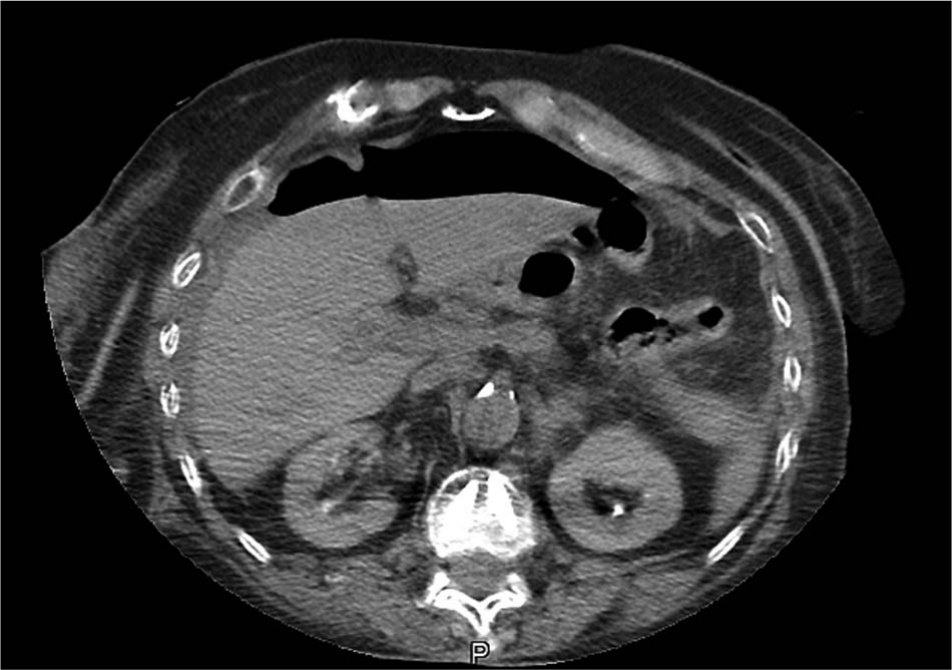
CT image of abdominal free gas.
